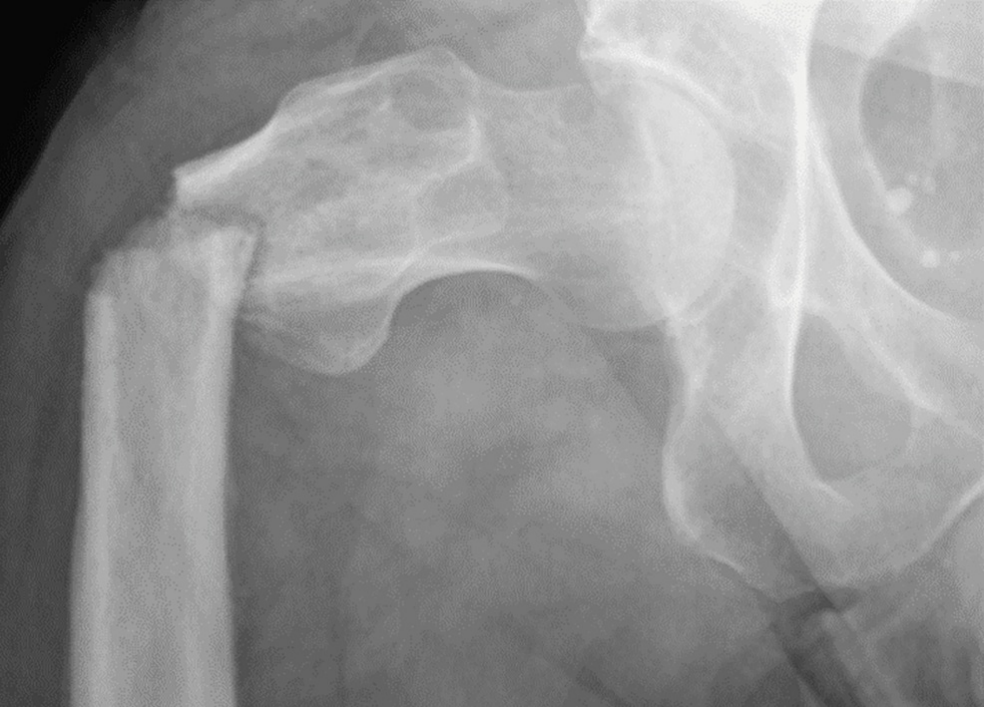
An AP radiograph taken on admission when the patient returned with the proximal femoral fracture AP: anteroposterior
